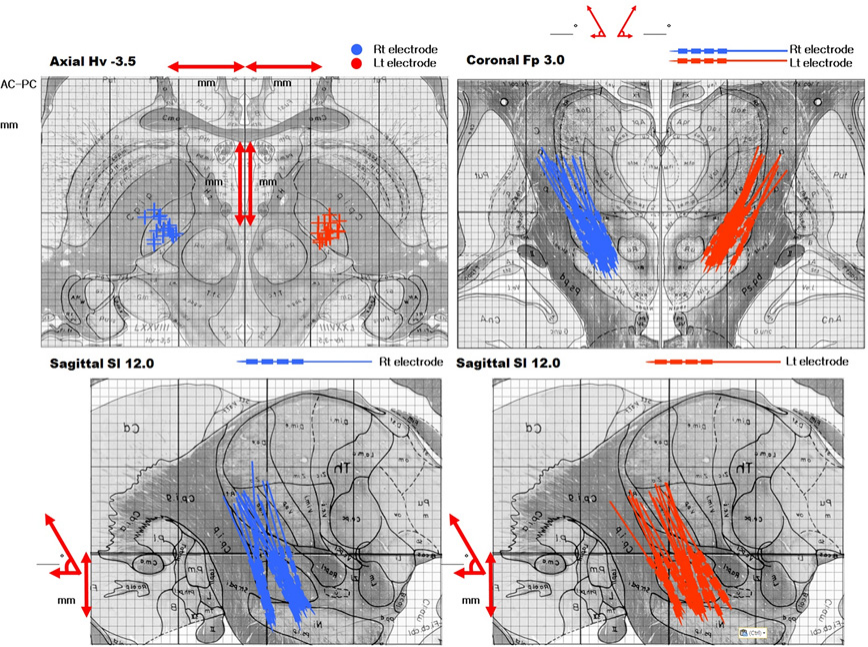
The location of electrodes on brain atlas. Based on the CT-MRI fusion images of the preoperative brain MRI and postoperative brain CT scan taken one month after surgery. The figure shows location of the electrodes plotted onto the human brain atlas of Schaltenbrand and Wahren.
